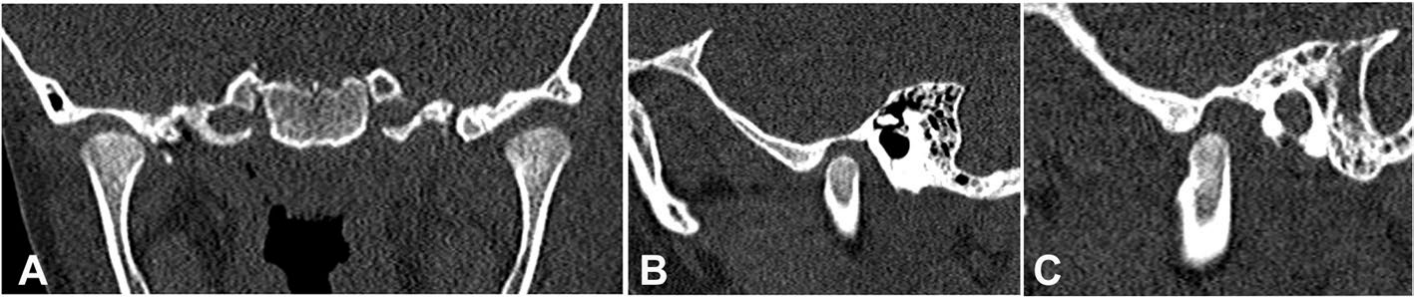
Images of maxillofacial computed tomography (CT) performed 2 years earlier showing both TMJs in coronal view (A), right TMJ in sagittal view (B) and left TMJ in sagittal view (C)
